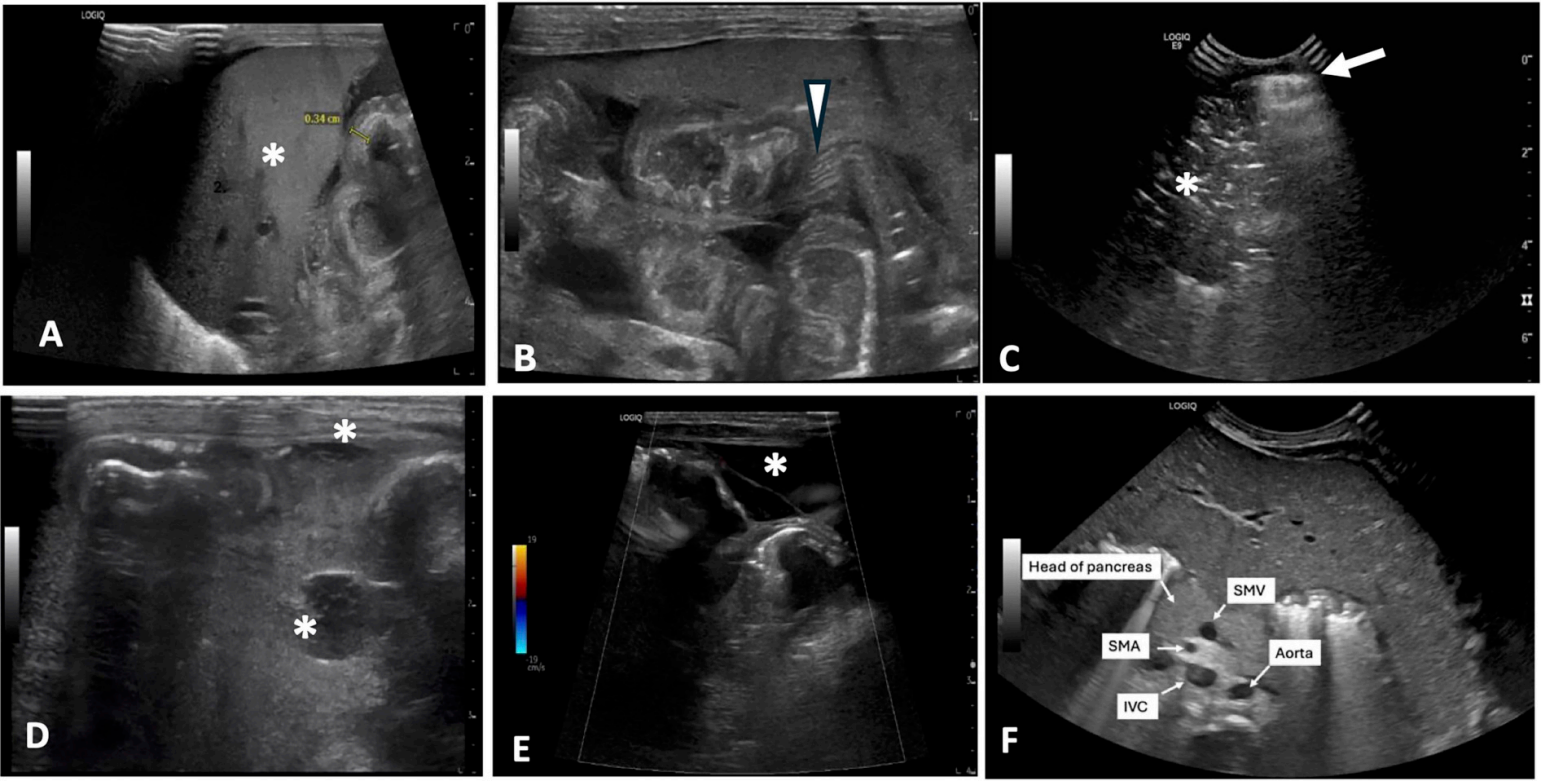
Representative AUS images of select HRF. (A) Thickened, echogenic bowel measuring 0.34 cm near liver (*). (B) Echogenic bowel wall with thickening of bowel valvulae conniventes and their hypoechoic interspaces resembling zebra stripe pattern (arrowhead). (C) Pneumoperitoneum seen as a bright echogenic stripe with reverberation artifact (arrow) just above anterior aspect of liver. Portal venous gas is also present (*). (D) Complex fluid collections (*). (E) Segment of bowel with diminished perfusion as seen on color Doppler imaging. Free fluid with septation is also seen surrounding bowel (*). (F) Reversal of SMV/SMA orientation with the SMV positioned to the left of the SMA. AUS, abdominal ultrasound; HRF, high risk findings; SMV, superior mesenteric vein; SMA, superior mesenteric artery; IVC, inferior vena cava.
